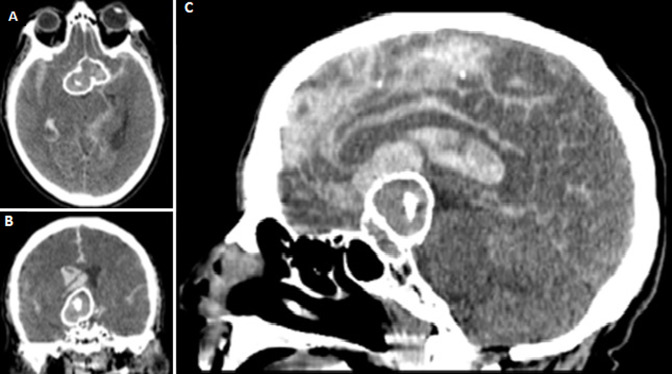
(A,B,C) brain computed tomography scan discovered a bilobed and bihemispheric huge mass (60 and 52 mm in diameter) of both frontal lobes with diffuse intraventricular hemorrhage
